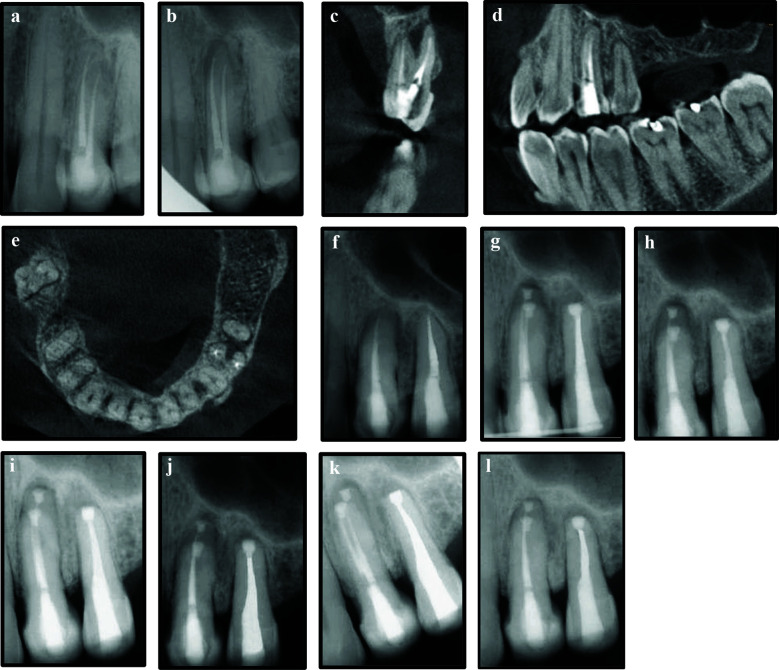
a, Periapical radiograph immediate post non-surgical endodontic treatment of tooth 24; b, Four years post-treatment; c, d & e, Cone beam computed tomographic cross-sectional, sagittal and axial views at the four year follow-up visit; f, Periapical radiograph immediate post non-surgical endodontic retreatment of tooth 24 and primary endodontic treatment of tooth 25; g, Intentional replantation of teeth 24 and 25; h, One month follow-up; i, Three months follow-up; j, Six months follow-up; k & l, 12 months and 16 months follow-up respectively.
